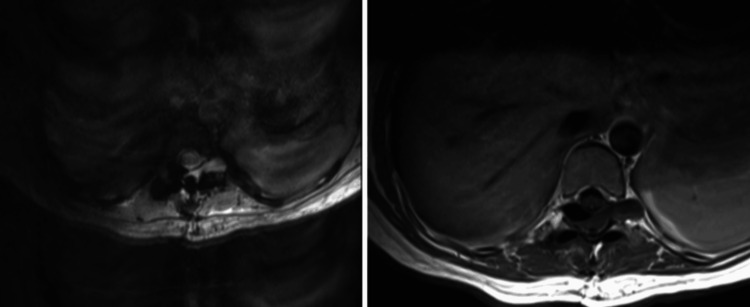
Postoperative axial T2-weighted MRI confirming absence of the cystic lesion and restoration of the spinal cord’s normal position, with no residual compression
